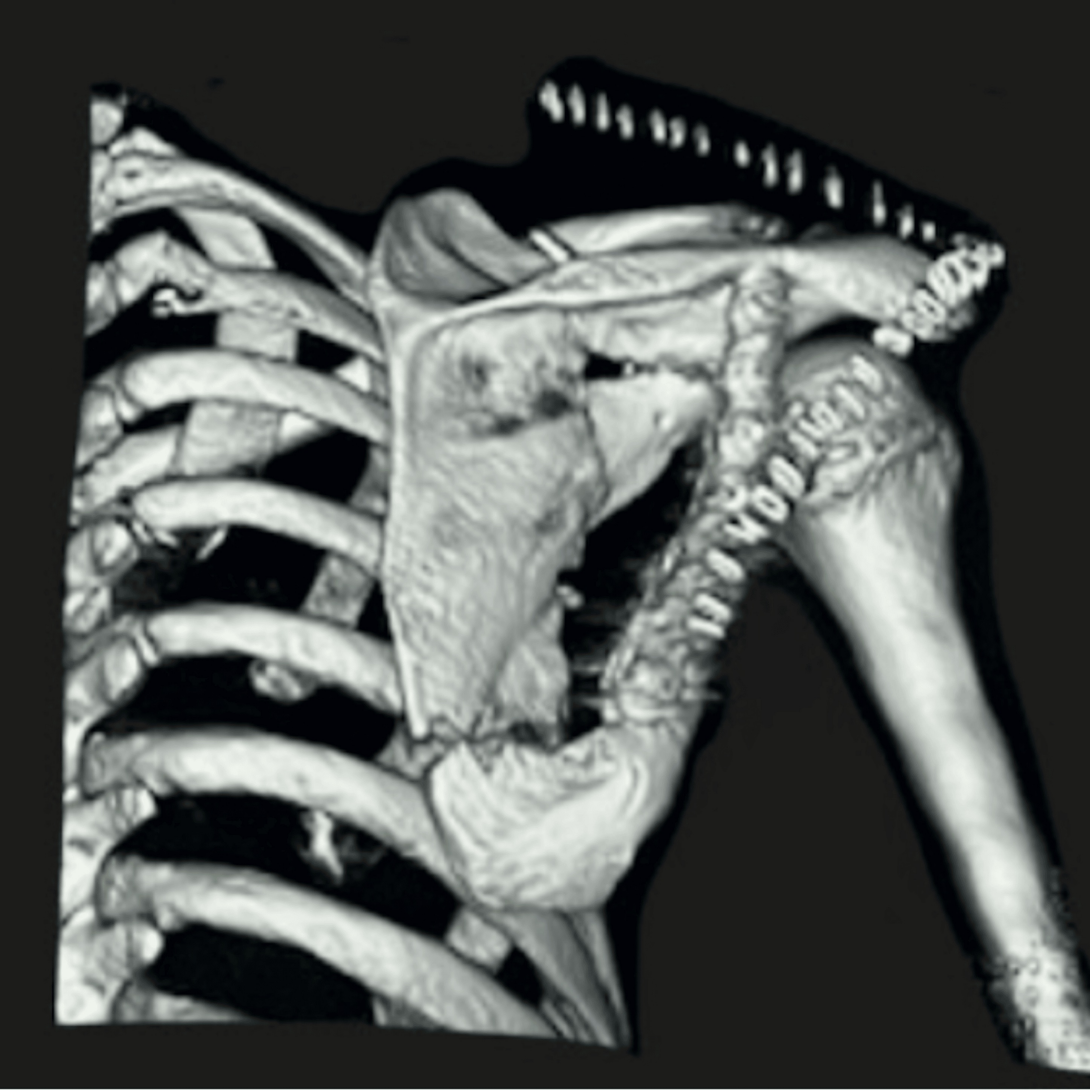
Postoperative CT bone reconstruction of the left shoulder. CT: computed tomography
